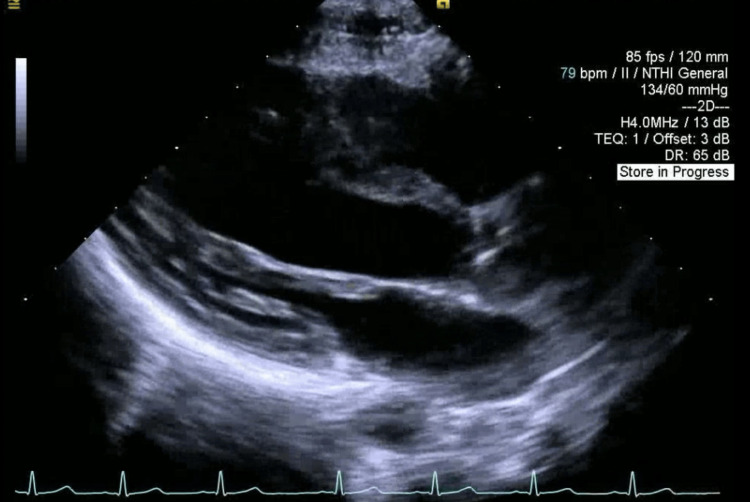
Transthoracic echocardiogram, parasternal long-axis view showing normal cardiac structure
